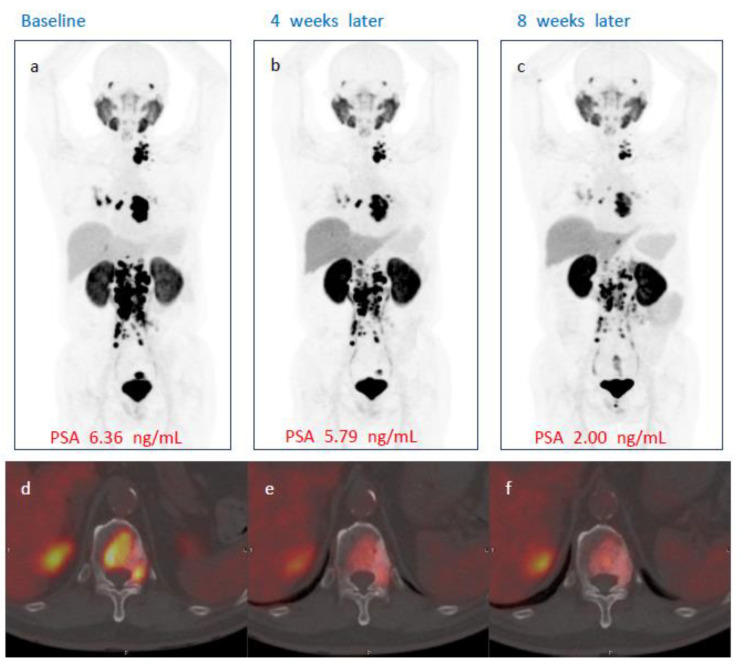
A case of a subject showing PSA response to [^177^Lu]Ludotadipep. (a,d) Baseline [^18^F]Florastamin PSMA PET/CT maximum intensity projection (MIP) and fused axial images with multiple bone and lymph node metastases. (b,c) PSMA PET/CT MIP images at four and eight weeks after administration of [^177^Lu]Ludotadipep showing decreased tumor burden. (e,f) Axial fused PSMA PET/CT images at four and eight weeks show decreased uptake in metastatic lesion in the thoracic vertebra. The patient’s PSA decreased from 6.36 to 2.00 ng/mL at 8 weeks.
